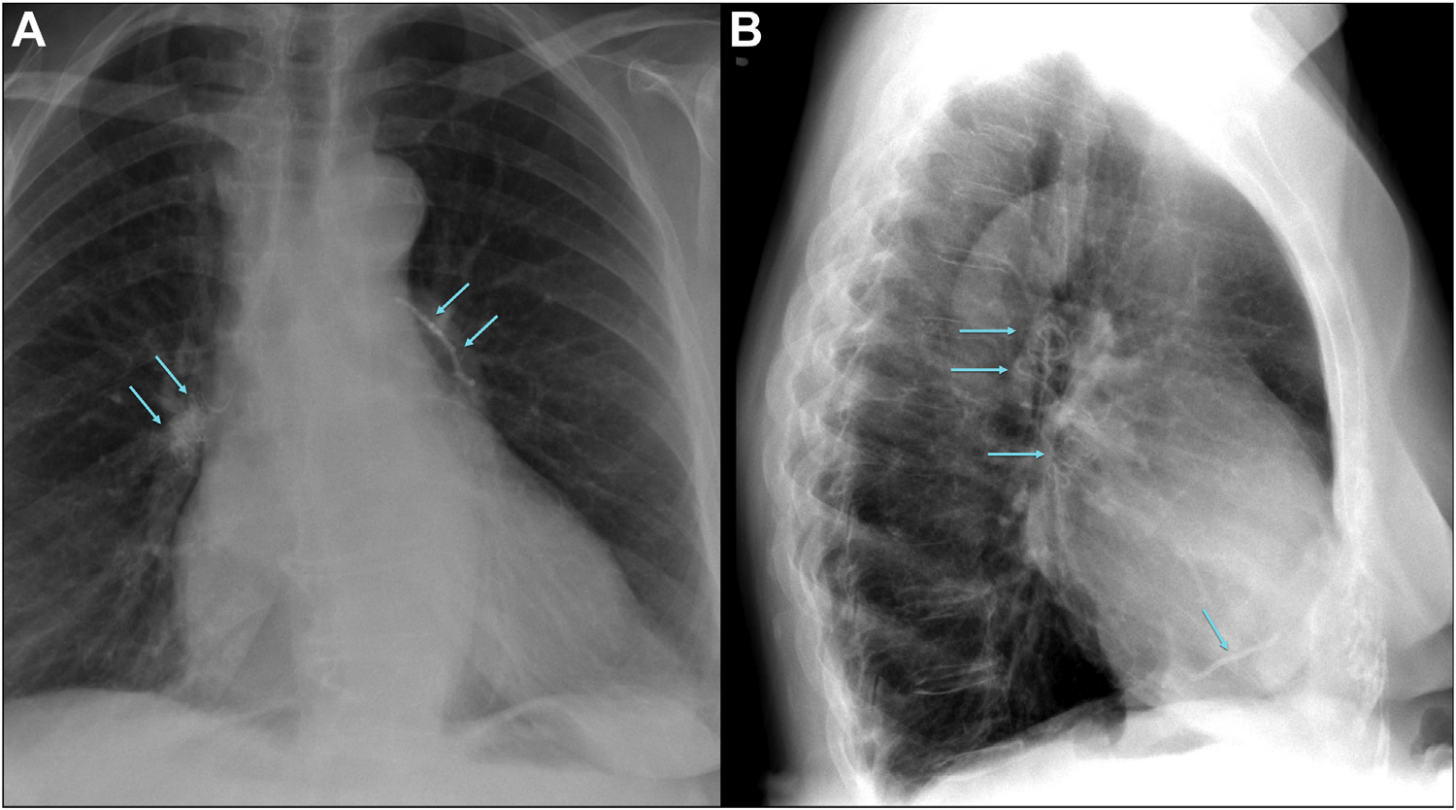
Conventional Chest X-Ray Cement emboli (arrows) in the pulmonary arteries and the right ventricle in posterior anterior (A) and lateral (B) view.
